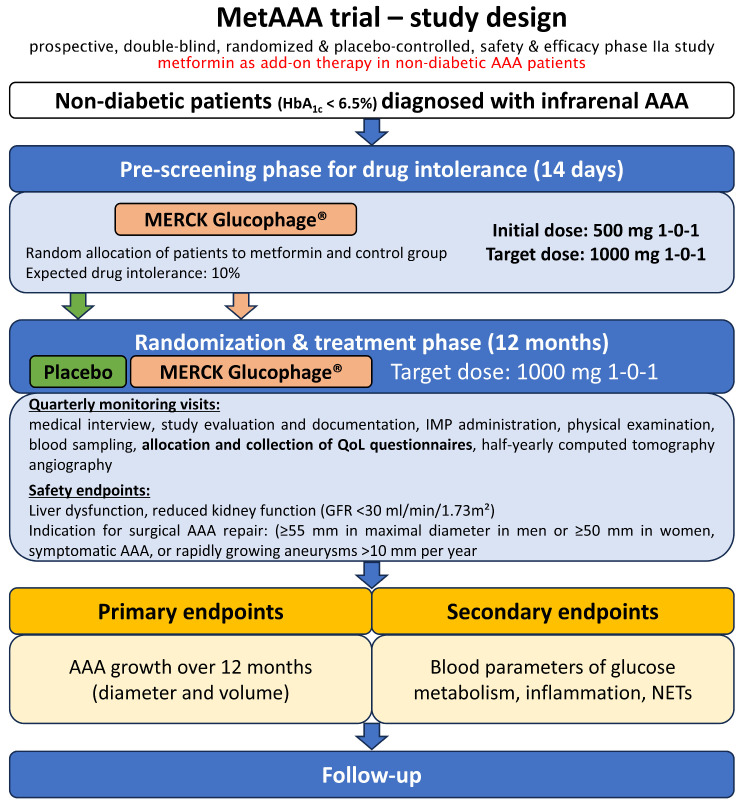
Overview of the MetAAA trial study design. Adapted from Klopf et al. [13]. In the MetAAA trial, metformin was investigated in a prospective, randomized, double-blind, placebo-controlled study to evaluate its safety and therapeutic efficacy. Patients identified with an infrarenal AAA measuring 30–49 mm on computed tomography angiography were enrolled following the screening phase and subsequently assigned to treatment with either metformin or placebo. Quarterly monitoring visits during the twelve months of treatment and subsequent half-yearly follow-up visits were additionally used to allocate and collect established and validated questionnaires for the assessment of QoL. Abbreviations: AAA, abdominal aortic aneurysm; IMP, investigational medicinal product, QoL, quality of life; GFR, glomerular filtration rate; NETs, neutrophil extracellular traps.
